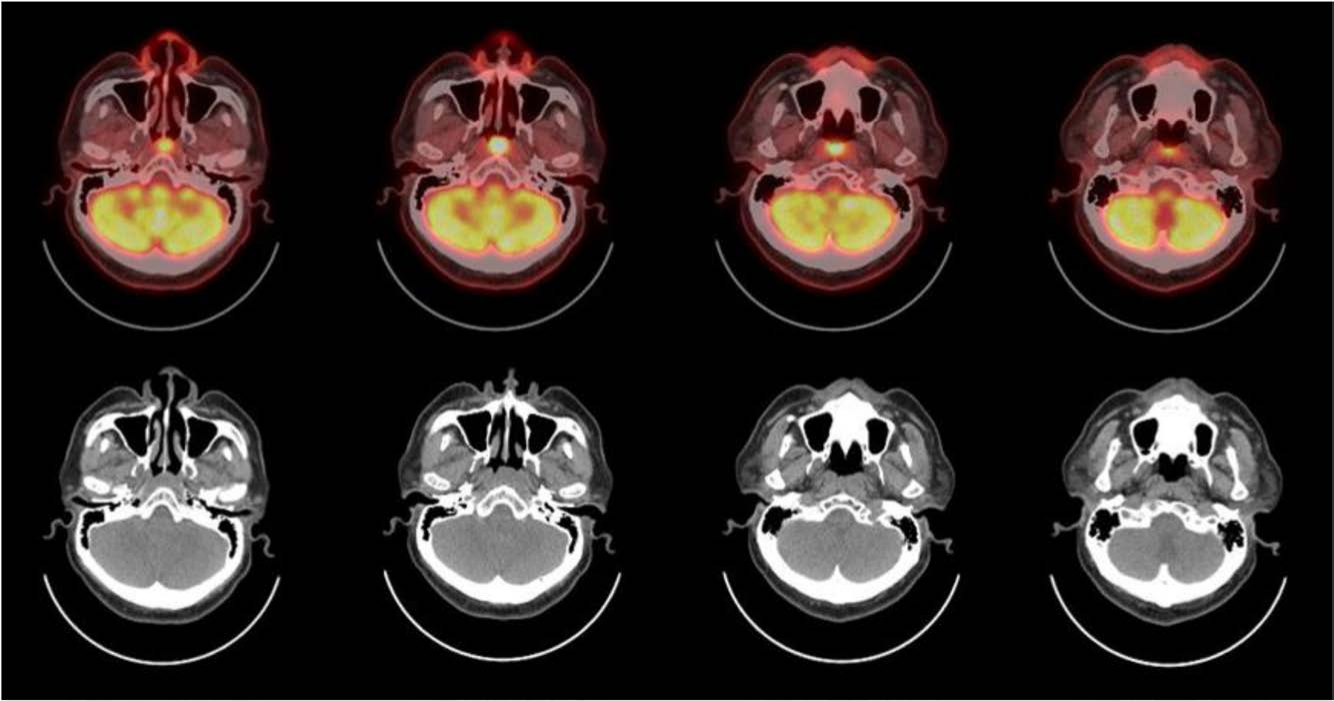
Axial ^18^F-FDG PET and PET/CT images in a 64-year-old man with nasopharyngeal tuberculosis. Pretreatment images show an abnormal ^18^F-FDG uptake on initial PET/CT over the nasopharynx, posterior wall. Malignant lesions could not be excluded and tissue confirmation was recommended. ^18^F-FDG = ^18^F-fluorodeoxyglucose, PET/CT = positron emission tomography/computed tomography.
